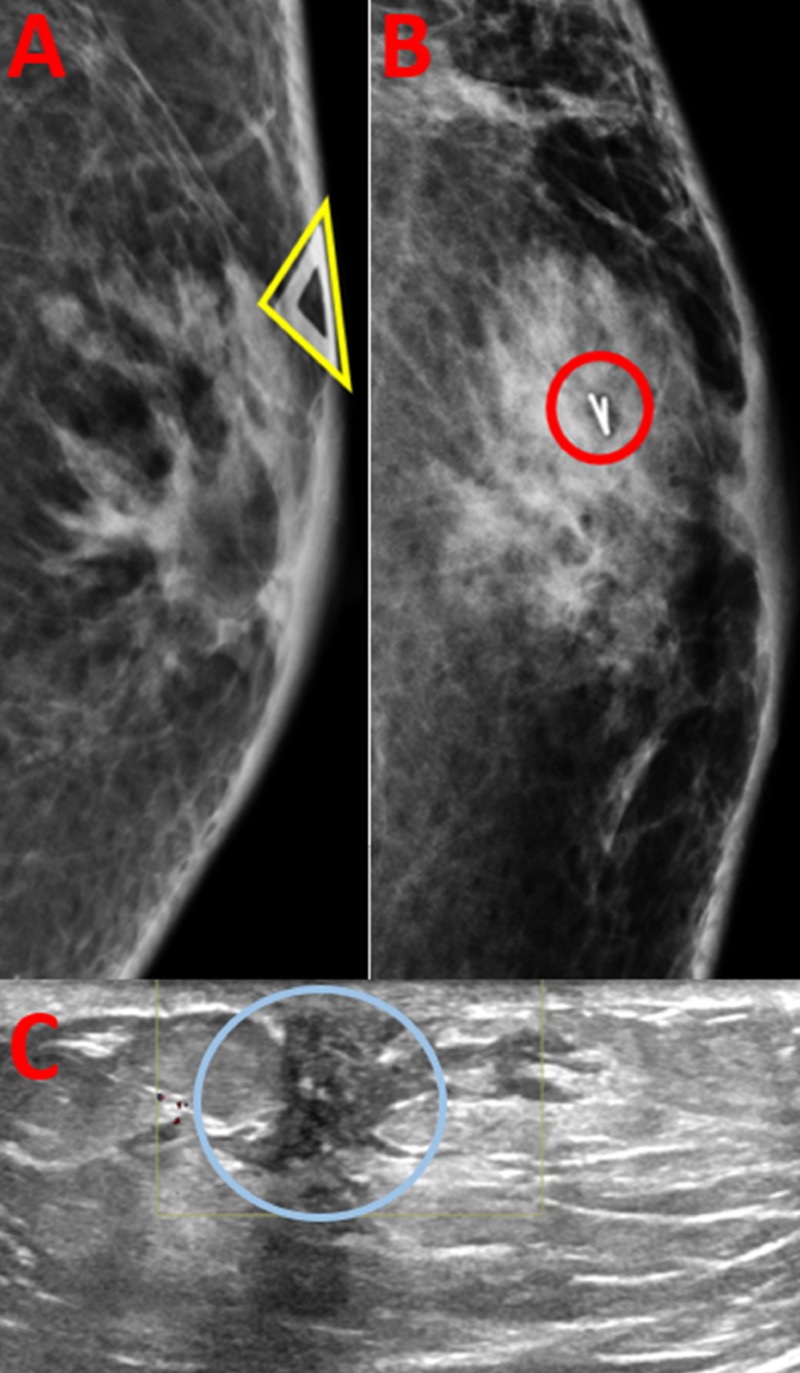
Imaging Findings of Pseudoangiomatous Stromal Hyperplasia (A) A diagnostic mammogram of left breast shows a subareolar focal asymmetry at the site of palpable marker (yellow triangle). (B) A post-biopsy mammogram of left breast demonstrates a ribbon biopsy marker clip within the biopsied mass (red circle), in the subareolar region. (C) A diagnostic ultrasound of left breast shows a hypoechoic irregular mass with angular margins (blue circle) with no internal vascularity, measuring 9 millimeters in the subareolar 12 o’clock region.
